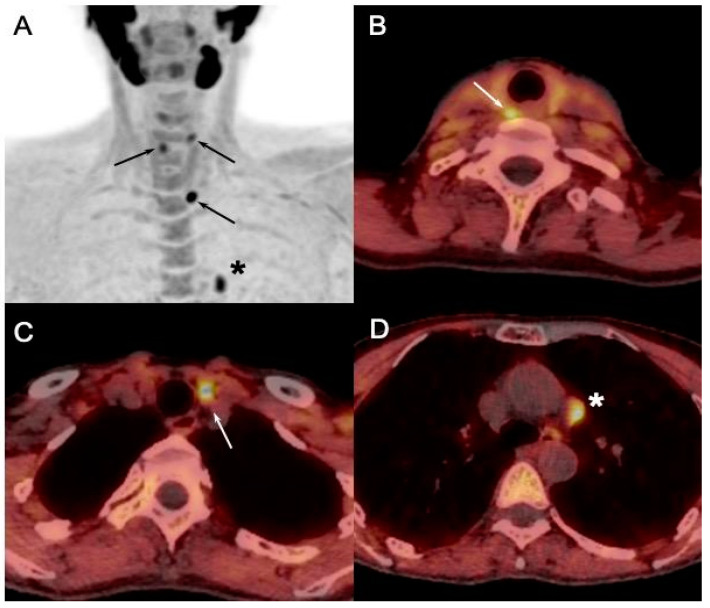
Initial staging in MEN1 patient with primary hyperparathyroidism (PTH: 157 ng/L; calcemia: 2.78 mmol/L) and probable left inferior parathyroid adenoma at cervical US. [^18^F]FCH PET/CT ((A): anterior MIP; (B–D): axial fusion images) confirmed US findings and detected two additional pathological parathyroids (arrows, superior right and left). In addition, hypermetabolic soft tissue nodule (*) of about 10mm was detected in left mediastinum, which suggested pathologic ectopic parathyroid, but turned out to be thymoma (B2) after surgery.
